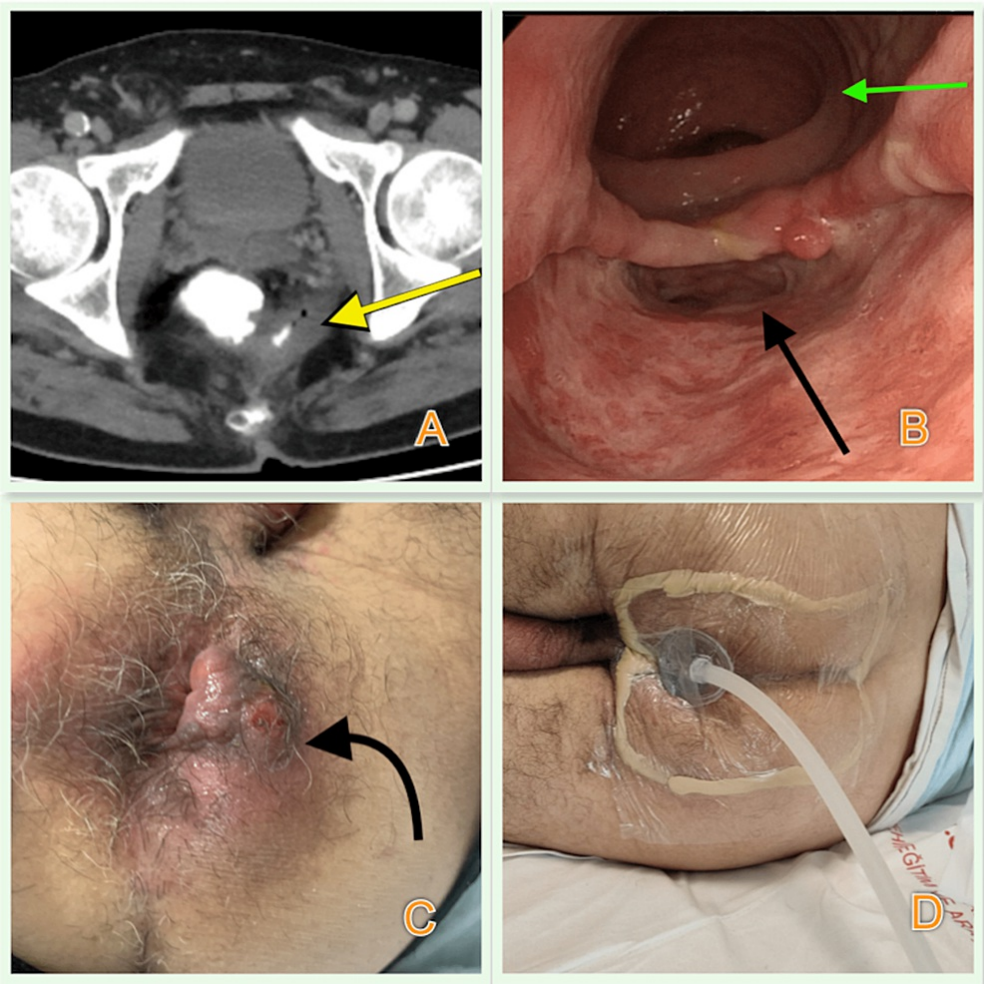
View of initial imaging studies, clinical presentation of the patient, and the demonstration of EVT A) CT view of ERP involving the posterolateral wall of the rectum (marked with yellow arrow); B) The view of rectal perforation on colonoscopy (the perforation area is marked with black and normal lumen is marked with green arrow); C) The view of perianal abscess in lithotomy position; D) EVT application with open-pore polyurethane sponge EVT: Endoluminal vacuum therapy; ERP: Extraperitoneal rectal perforation
